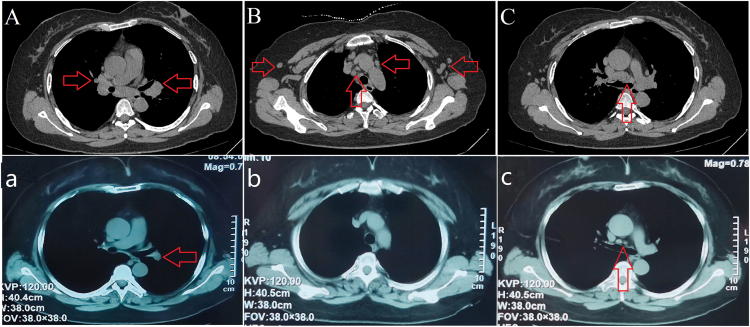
Computed tomography findings of the patient. At admission, multiple enlarged lymph nodes were observed in (A) both hilar regions, (B) supraclavicular and axillary areas, and (C) the mediastinum. After a 15-month follow-up, the (a) hilar lymph nodes and (c) mediastinal lymph nodes had significantly reduced, whereas (b) the lymph nodes at other locations had resolved.
